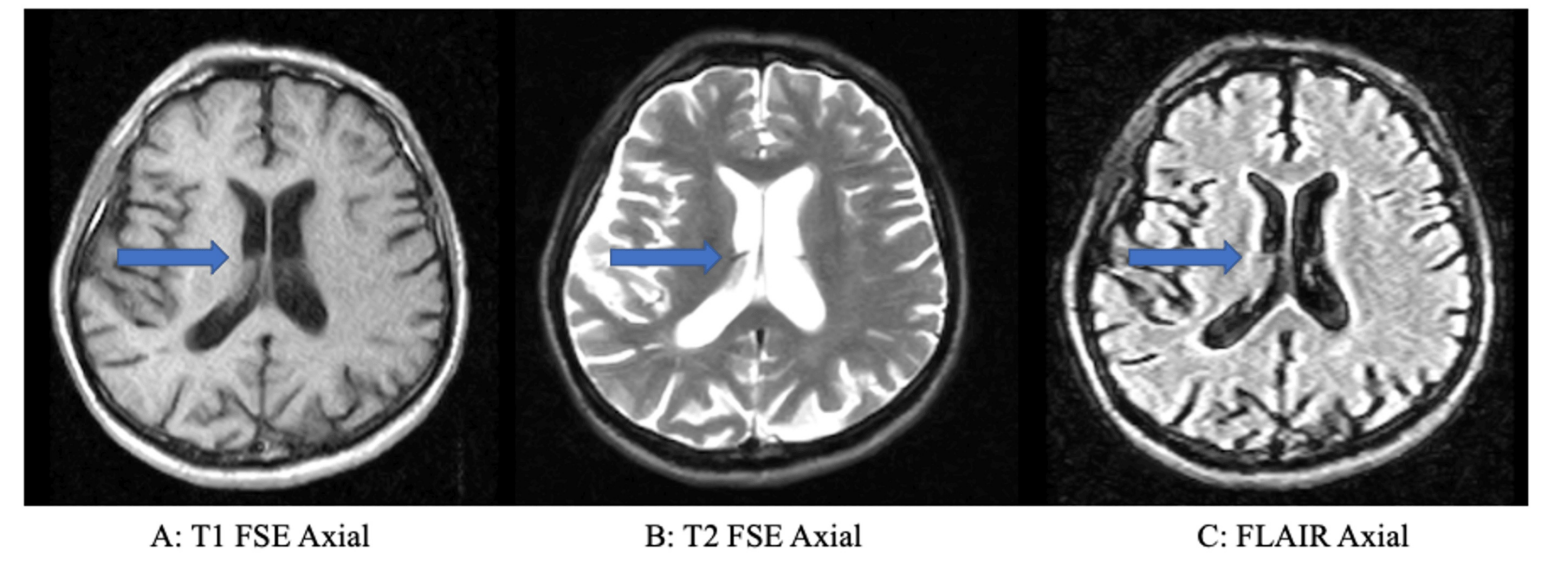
MRI of the lateral ventricles MRI: Magnetic resonance imaging; FSE: fast spin-echo; FLAIR: fluid-attenuated inversion recovery This figure depicts the MRI of the patient's lateral ventricles, indicated by the arrows. There was sinus disease and involutional changes with increased size of the lateral ventricles. A shows a T1 FSE axial view, B shows a T2 FSE axial view, and C shows the lateral ventricles in a FLAIR axial view
